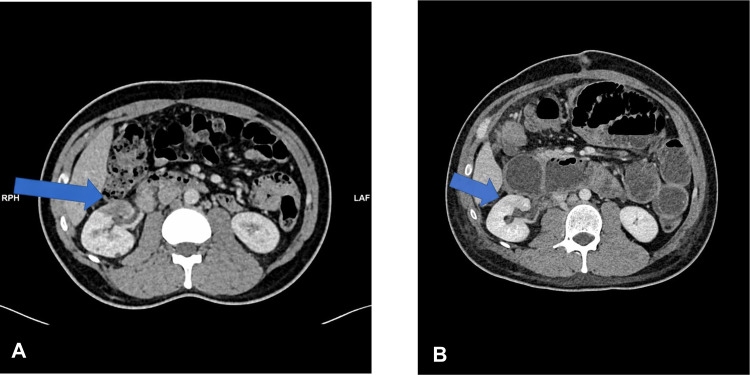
(A, B) – The first CT showing the right renal infarct, and the CT after surgery showing almost complete resolution of the infarct.
